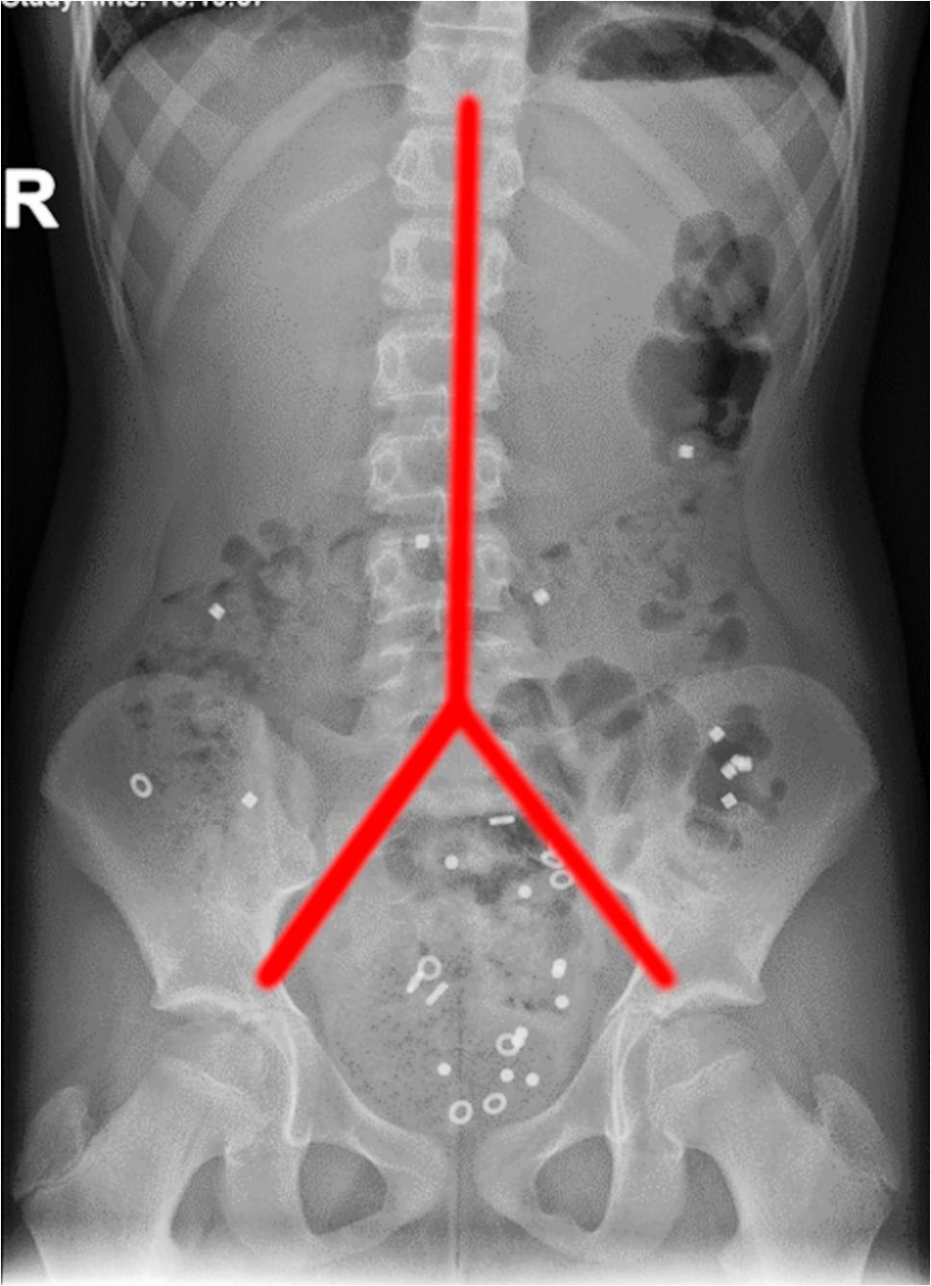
An anteroposterior abdominal radiograph in an 8-year-old girl with constipation referred for colon transit time (CTT) estimation. Markers were given for six days and the radiograph taken on day 7. For evaluation, the abdominal area is divided into three parts (*red lines*). There are four markers in the right quadrant, seven in the left quadrant, and 19 in the pelvic area. The transit times can be calculated by multiplying the markers by a factor of 2.4. Therefore, the transit time fare 9.6 h, 16.8 h, and 45.6 h for the right hemi-colon, left hemi-colon and recto-sigmoid, respectively, resulting in a total CCT of 72 h. Values for the right and left hemi-colon are just above the normal limits, while the time for the recto-sigmoid colon and total CTT are prolonged. The final diagnosis was functional constipation
